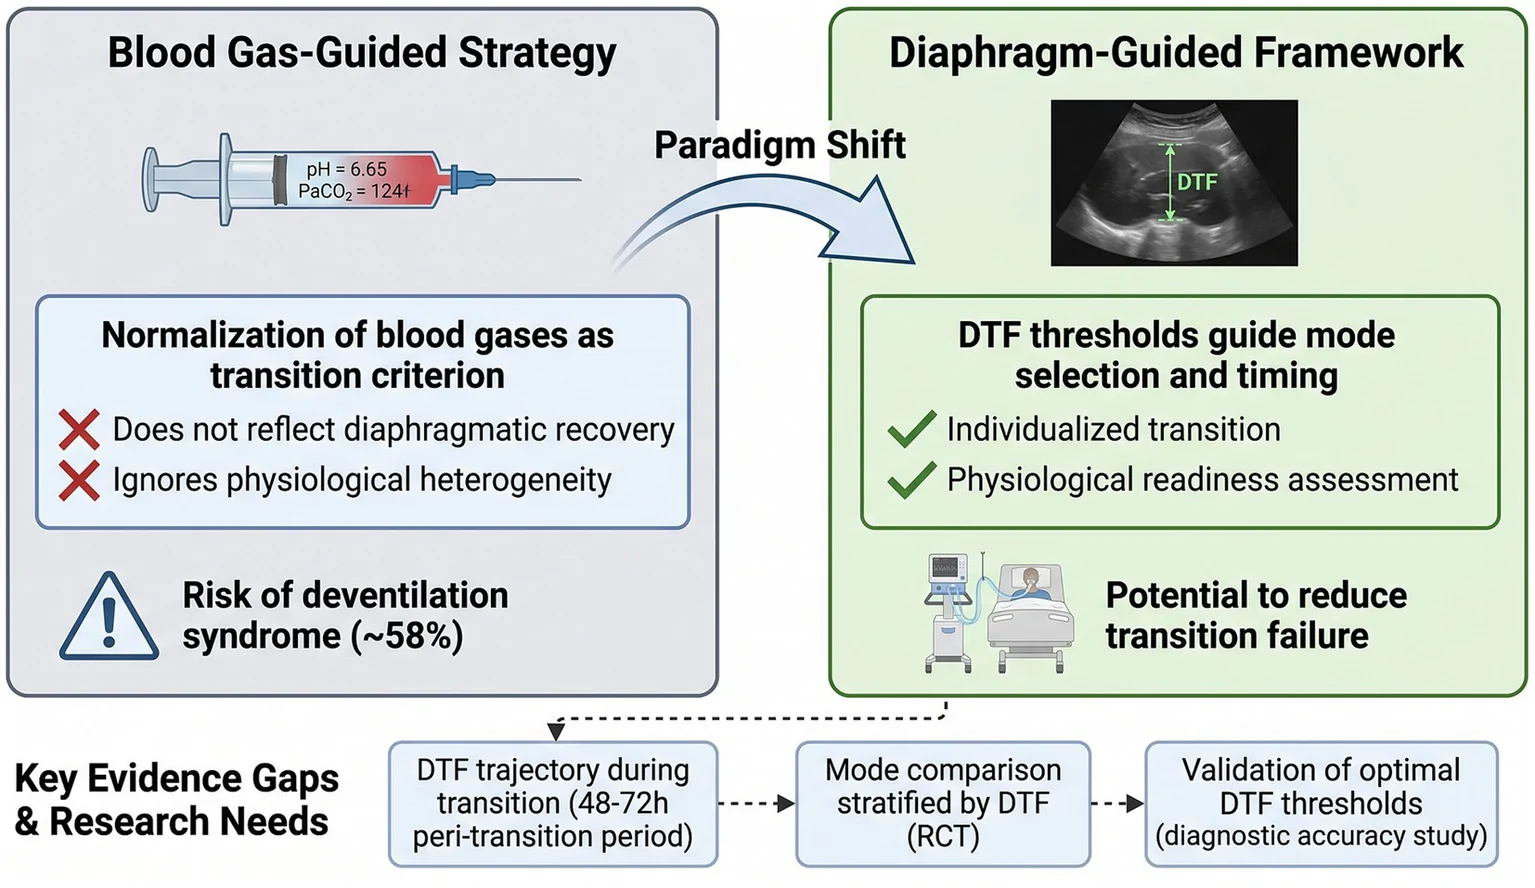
Paradigm shift from blood gas-guided to diaphragm-guided sequential therapy in COPD with type II respiratory failure. Left panel (traditional approach). Clinical decision-making has relied primarily on normalization of blood gases (pH and PaCO₂) as the criterion for NIV withdrawal. However, this strategy has two major limitations: (i) normalization of blood gases does not equate to recovery of diaphragmatic function, and (ii) it fails to account for physiological heterogeneity among patients. Consequently, in one prospective single-centre cohort (*n* = 67), 58% of patients on long-term home NIV met the study’s definition of DVS after termination of nocturnal ventilation; reported prevalence varies substantially with the definition applied. Right panel (proposed diaphragm-guided framework). The framework uses serial diaphragmatic ultrasound measurements of DTF to assess diaphragmatic functional reserve. DTF thresholds (<20%, 20–30, >30%) guide mode selection (S/T, AVAPS, or PAV/NAVA) and transition timing, enabling individualized, physiology-based sequential therapy. Bottom panel (future directions). Three key evidence gaps require prospective validation: (i) the trajectory of DTF during the 48- to 72-h peri-transition period; (ii) mode comparison stratified by DTF in randomized controlled trials; and (iii) validation of optimal DTF thresholds in diagnostic accuracy studies. DVS, deventilation syndrome; DTF, diaphragmatic thickening fraction; NIV, noninvasive ventilation; RCT, randomized controlled trial.
